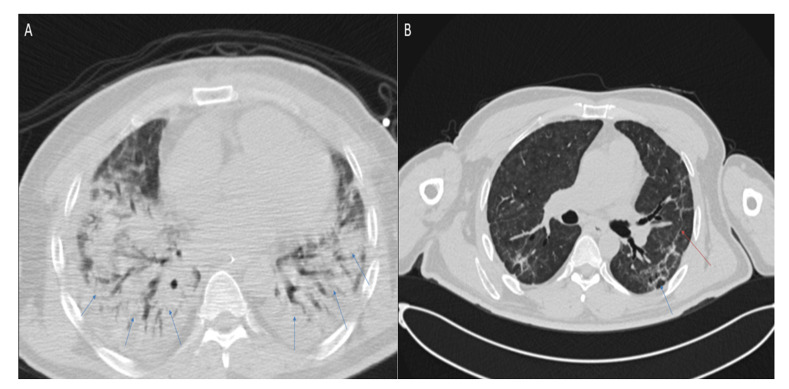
Axial chest CT images (lung window) of a 47-year-old patient obtained (A) at hospital admission with confirmed acute COVID-19 shows signs of bilateral polysegmental pneumonia and ground-glass opacities. Lung damage ~80%. Blue arrows indicate infiltration of the lung parenchyma; (B) six months after acute COVID-19 shows traction wall thickening of segmental and subsegmental bronchi in the parenchyma of both lungs. Fibrous cords, irregularly linear forms, without signs of infiltration, mediastinal lymphadenopathy. Fibrosis is marked with a red arrow, dilated bronchus with a blue arrow.
